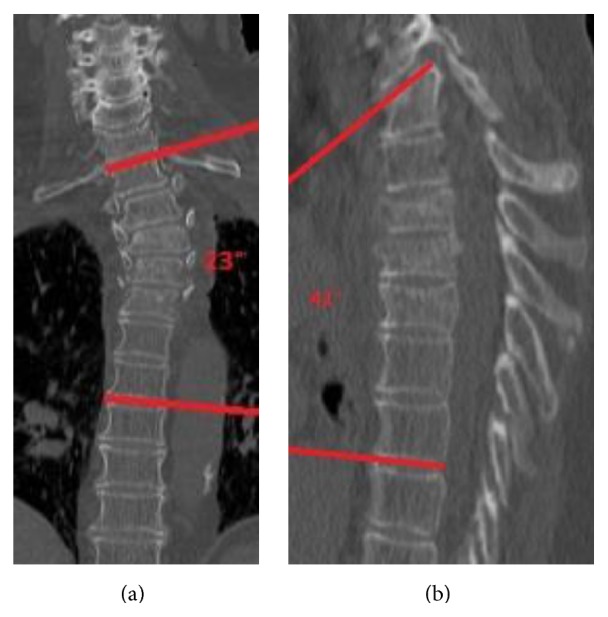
(a) CT scan showing the scoliosis and osteoporosis of the patient and the fractures in T3–T5. Cobb angle (T1–7): 23°. (b) CT scan in the sagittal profile. Kyphosis angle (T1–7): 41°.
